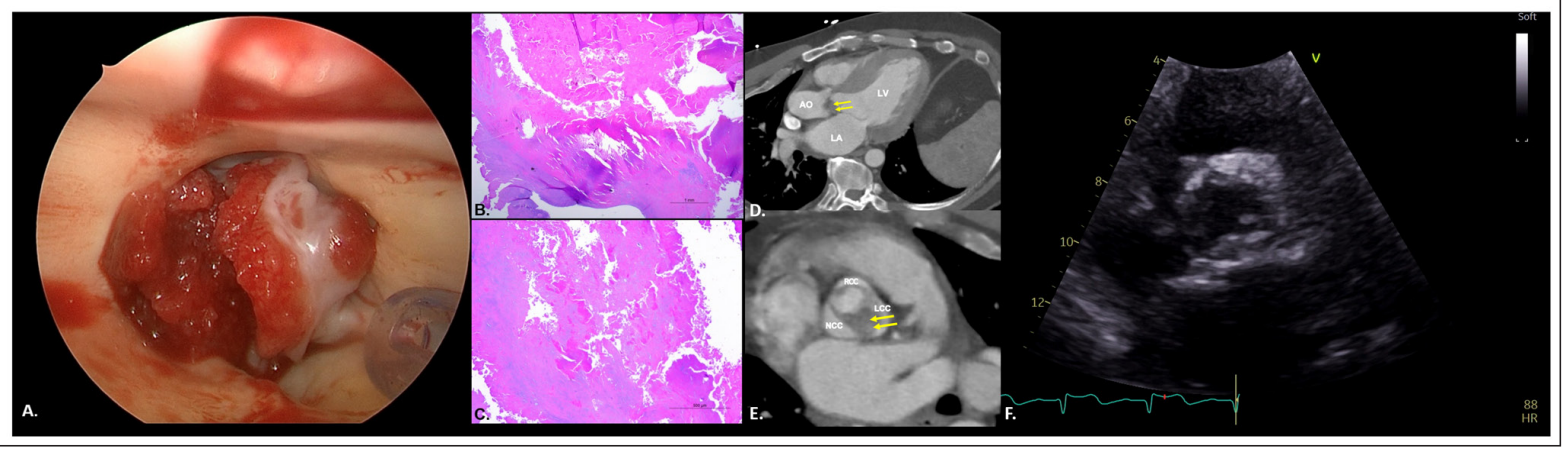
Marantic endocarditis. (A) Intraoperatively, the valve appears stenotic with a heavily calcified annulus and fresh-formed thrombus on both the outflow and inflow. (B) Low magnification of a vegetation composed of fibrin, forming an eosinophilic mass. Mature fibrosis is seen at the base of the vegetation. (C) High magnification shows that vegetation is composed predominantly of fibrin and red blood cells. (D) 3-chamber view on non-gated computed tomography shows ventricular hypertrophy and subvalvular aortic valve thickening extending to the left ventricular outflow tract (arrows). (E) Short axis of the aortic valve on non-gated computed tomography shows bicuspid aortic valve opening (Sievers type 0) with severe thickening of the left coronary cusp leaflet and no calcification (arrows). (F) Parasternal short axis view shows thickened aortic valve leaflets with systolic doming and restricted opening.
